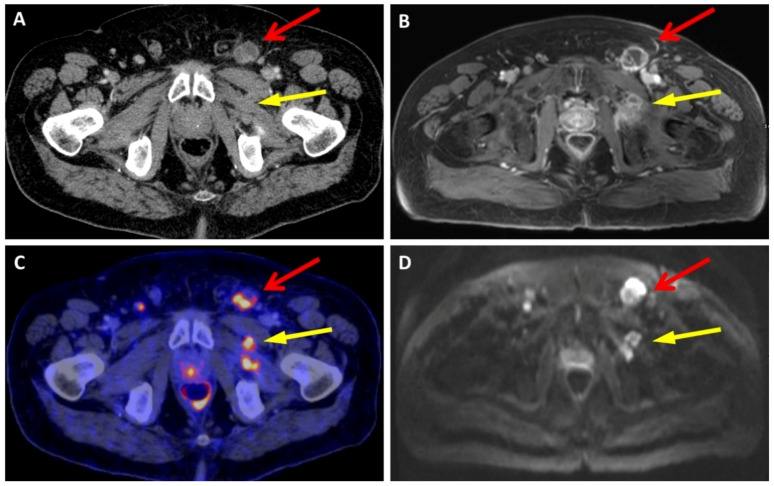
This patient exhibited metastatic lymph nodes in the left inguinal region (indicated by the red arrow) and metastatic soft-tissue lesions in the left M. obturatorius externus (highlighted by the yellow arrow), which could be distinguished using all imaging modalities. (A) is CT, (B) is VIBE, (C) is PET/CT, and (D) is HASTE.
